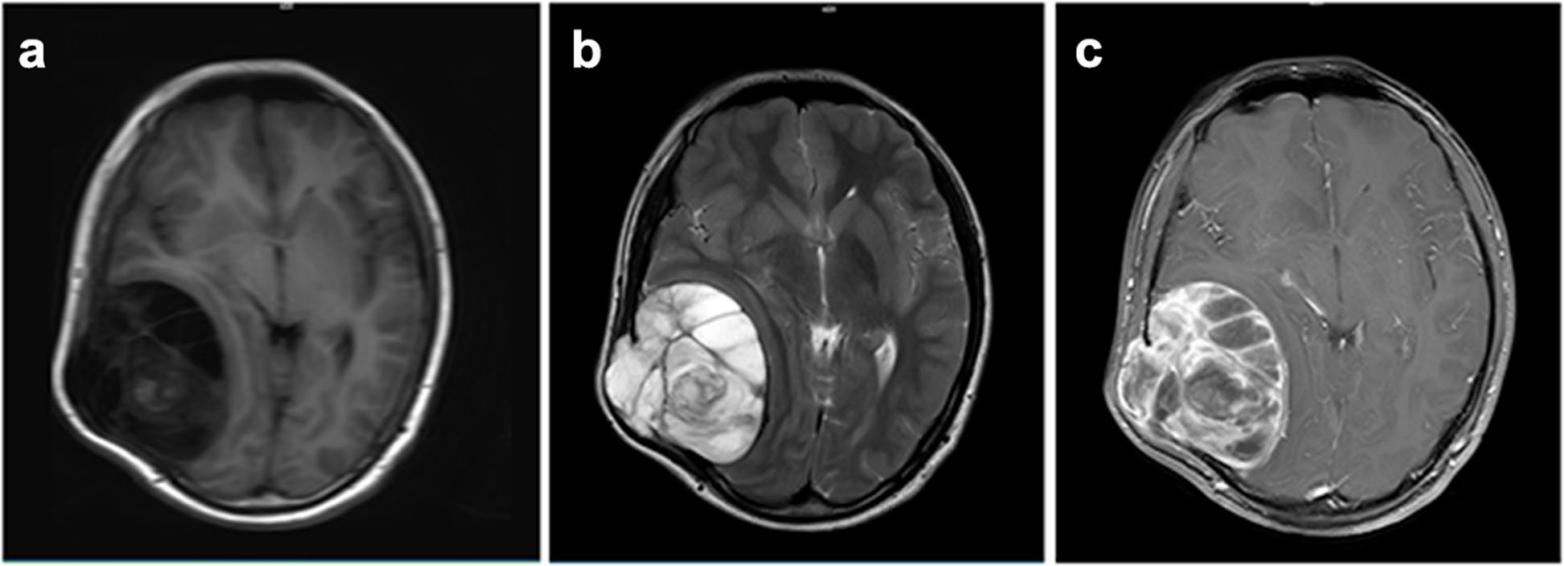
Axial head resonance imaging (MRI) (a-c) scan shows a right temporal mass with trabecular aspect inside and compressing temporal lobe (a-c). The mass mainly shows a signal similar to that of liquid on the T1(a) and T2 (b) weighted images. The mass shows marked contrast enhancement (c, T1-weighted fat-suppressed image after contrast enhancement)
